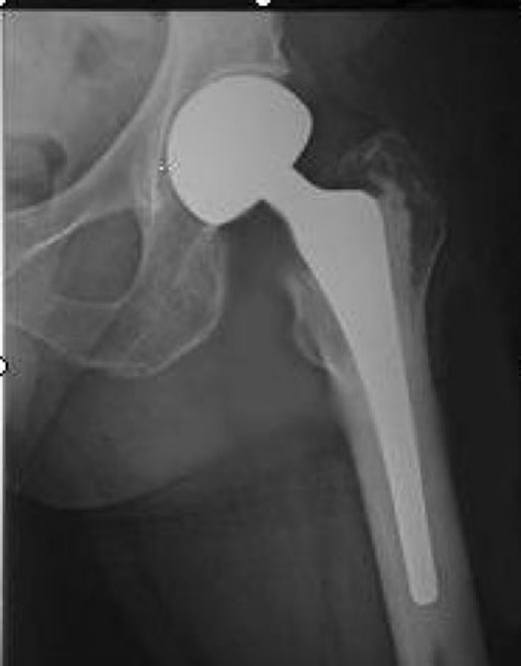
A 67-year-old patient with fracture neck femur treated with bipolar hip arthroplasty. At the final follow-up radiograph showing the outer head of the prosthesis in the anatomic position, articular cartilage space has been preserved, and neither migration of the outer head nor loosening of the femoral stem was seen
